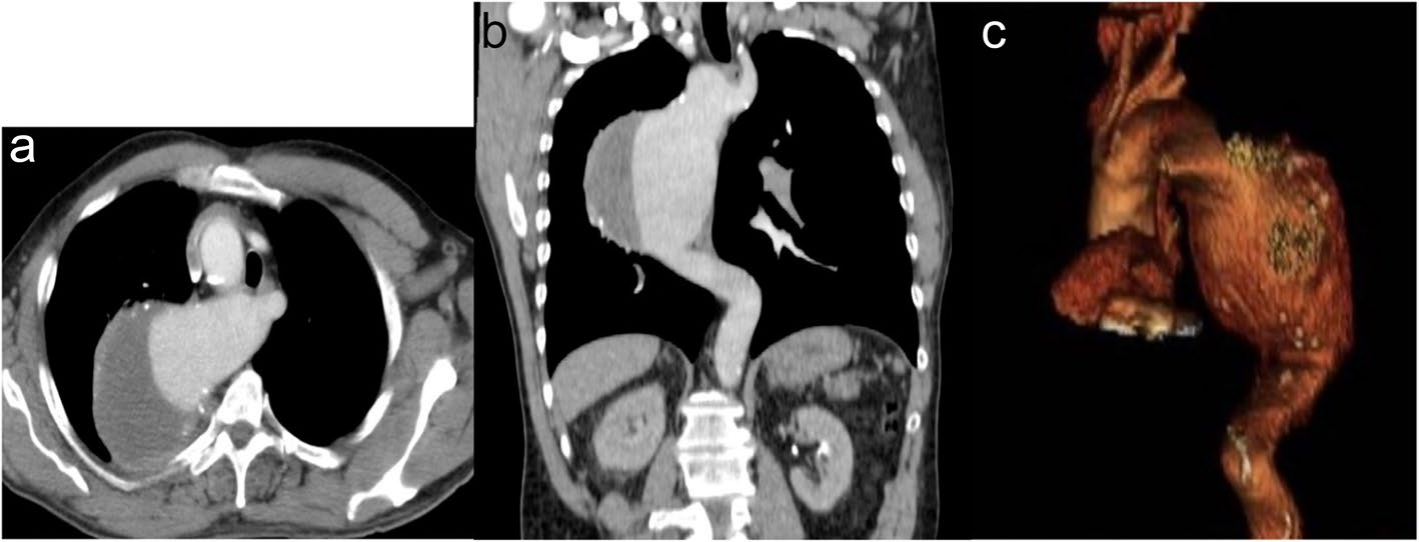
Preoperative CTA: a) axial reconstruction of right sided aortic arch and the origin of left common carotid artery and retroesophageal left subclavian artery b) coronal reconstruction of the aneurysm of descending aorta c) volume rendering
